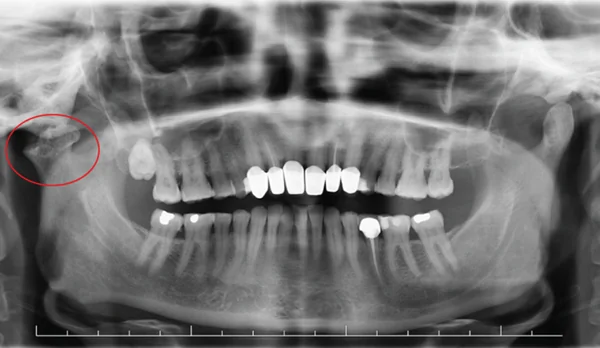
Panoramic radiograph demonstrating the incidental finding of a multilobulated right mandibular condyle.
